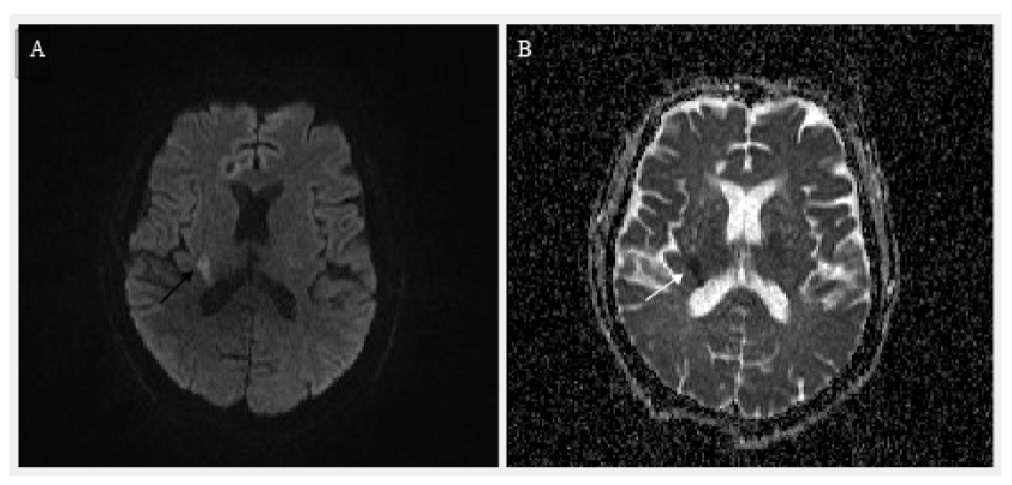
MRI of the brain demonstrating acute infarct. (A) MRI demonstrates hyperintensity within the right internal capsule on diffusion weighted images (black arrow). (B) Acute infarction is confirmed by hypointensity within the same territory on apparent diffusion coefficient (white arrow).
